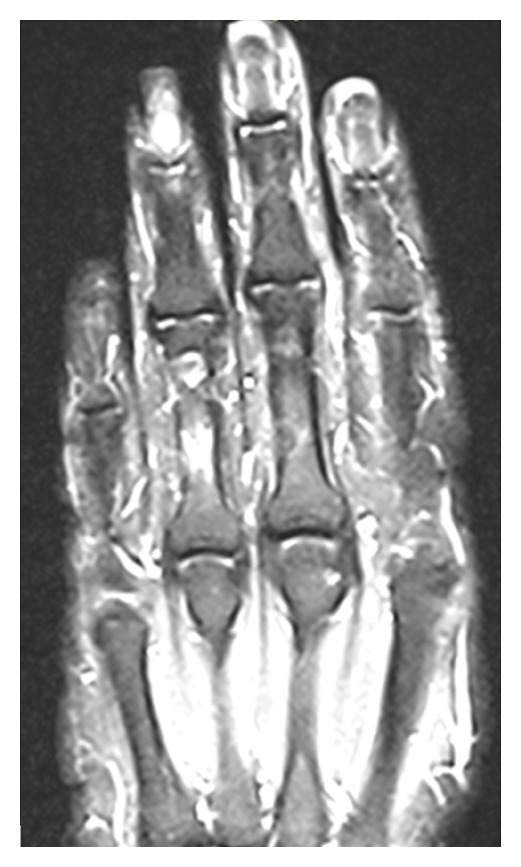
MRI of right hand showing glomus tumor of 4th digit.
